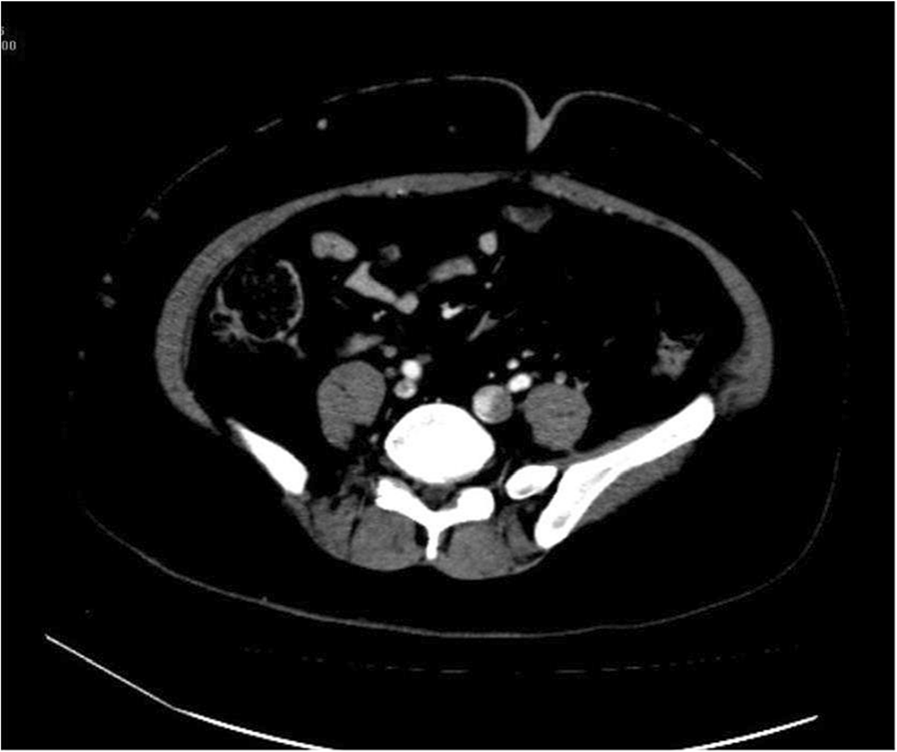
Computed tomography scan below the level of the kidneys showing a filling defect in the inferior vena cava.
